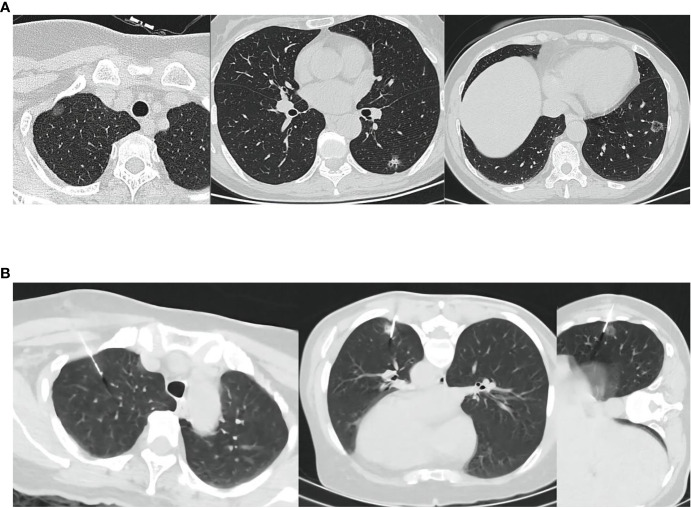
(A) The location of the lesion shown on CT. (B) Indocyanine green was injected intravenously and punctured under CT. CT, Computed tomography; ICG, indocyanine green.
